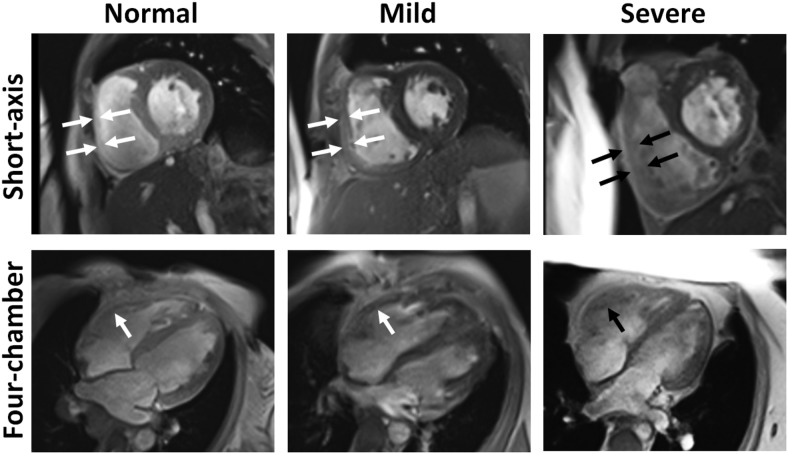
Right ventricular (RV) hypertrophy in pulmonary arterial hypertension shown on cine MR images. Short-axis (top) and the corresponding 4-chamber (bottom) slices of a patient without hypertrophy (left) and patients with mild (middle) and severe (right) RV hypertrophy. Note the thick RV wall, which is translated into increased RV mass.
